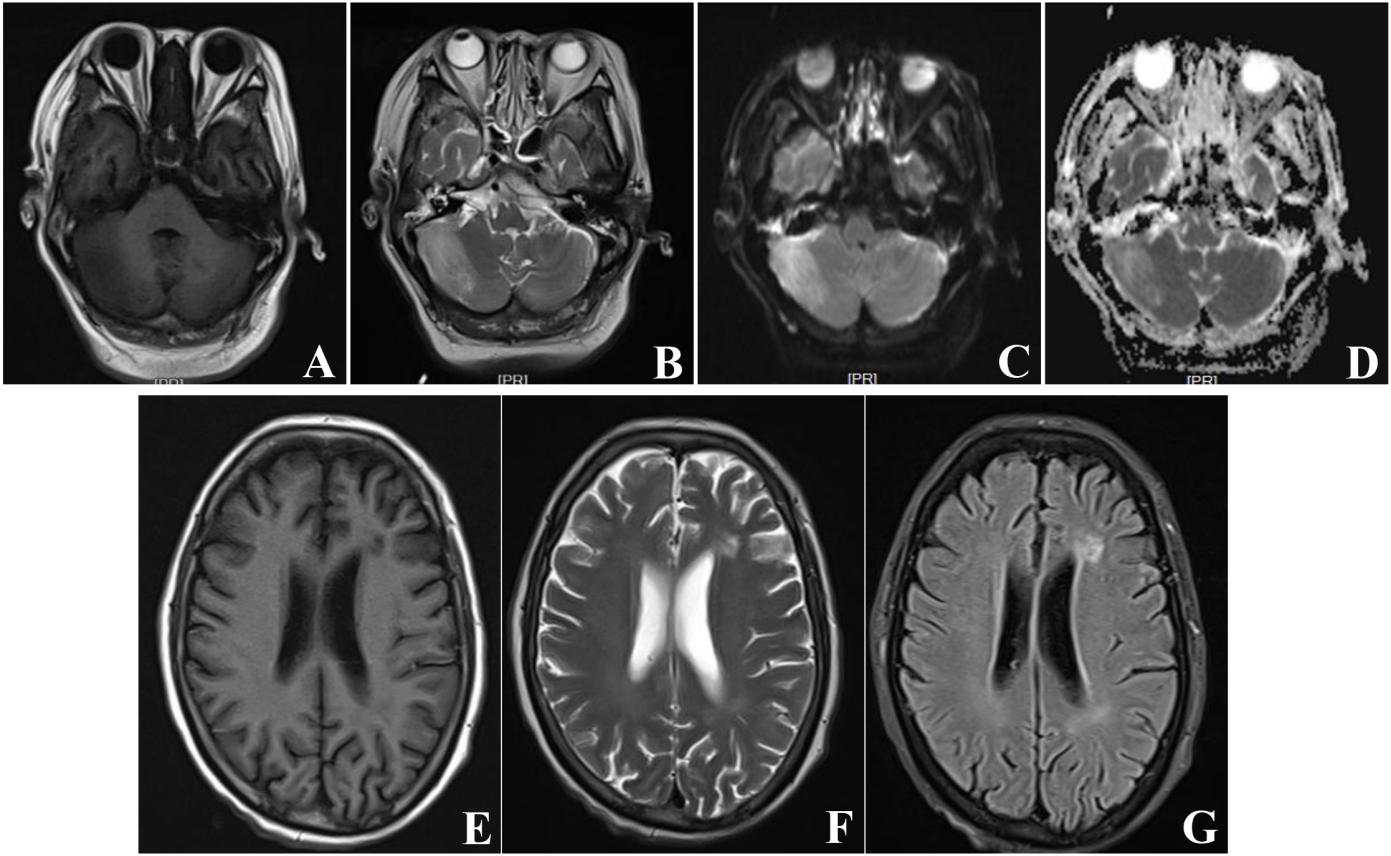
MRI images. MRI was performed after treatment. The MRI images showed the right cerebellum a patchy long T1 (A) and long T2 (B) signal shadow, with equivalent signal intensity on DWI (C) and a slightly longer signal on ADC (D), and the left insular lobe a patchy long T1 (E) and slightly long T2 (F) signal shadow, with high signal on T2 Flair imaging (G).
